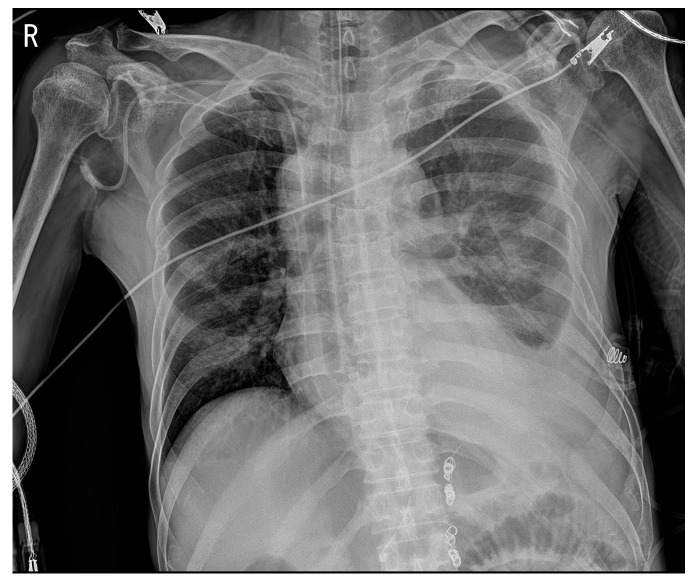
Anteroposterior chest X-ray after Sengstaken–Blakemore tube insertion showing the esophageal balloon placement at 24 cm depth.
